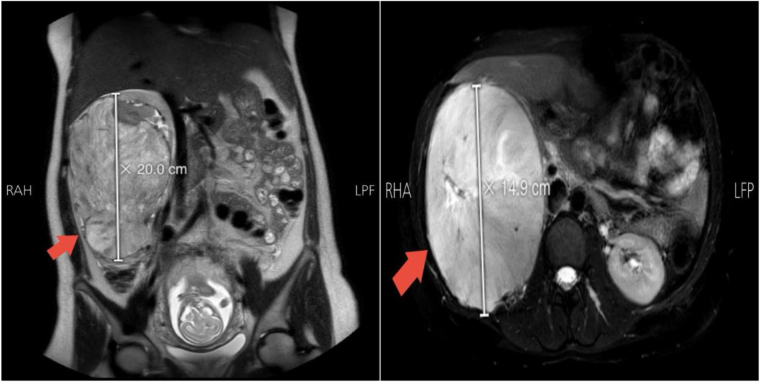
MRI showing a large right renal mass measuring approximately 20.0 cm in height (left) and 14.9 cm in width (right).
